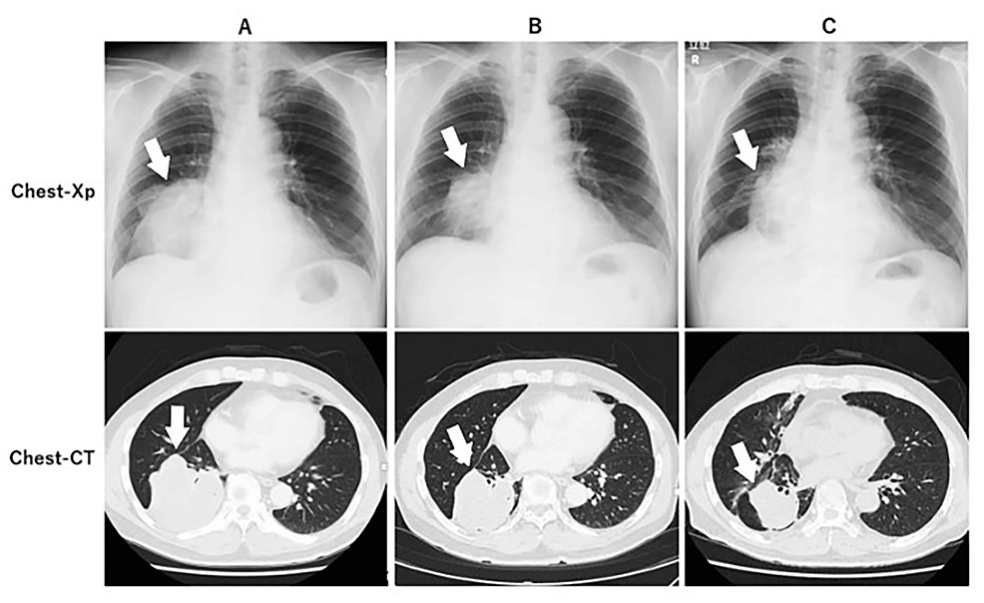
Lung cancer changes in chest Xp and CT A: - 16 days. B: 29 days. C: 130 days. Signs of radiation pneumonitis around the lung cancer. The white arrows indicate cancer.
